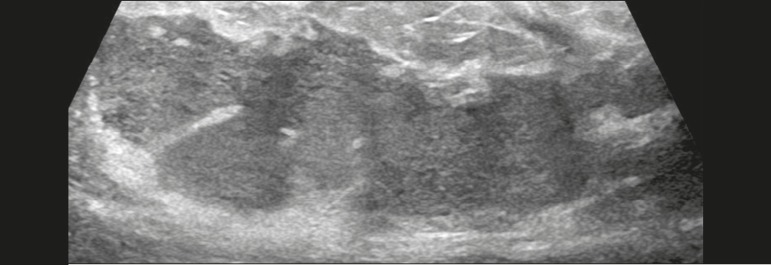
Ultrasound of a 35-year-old female patient who presented with an
